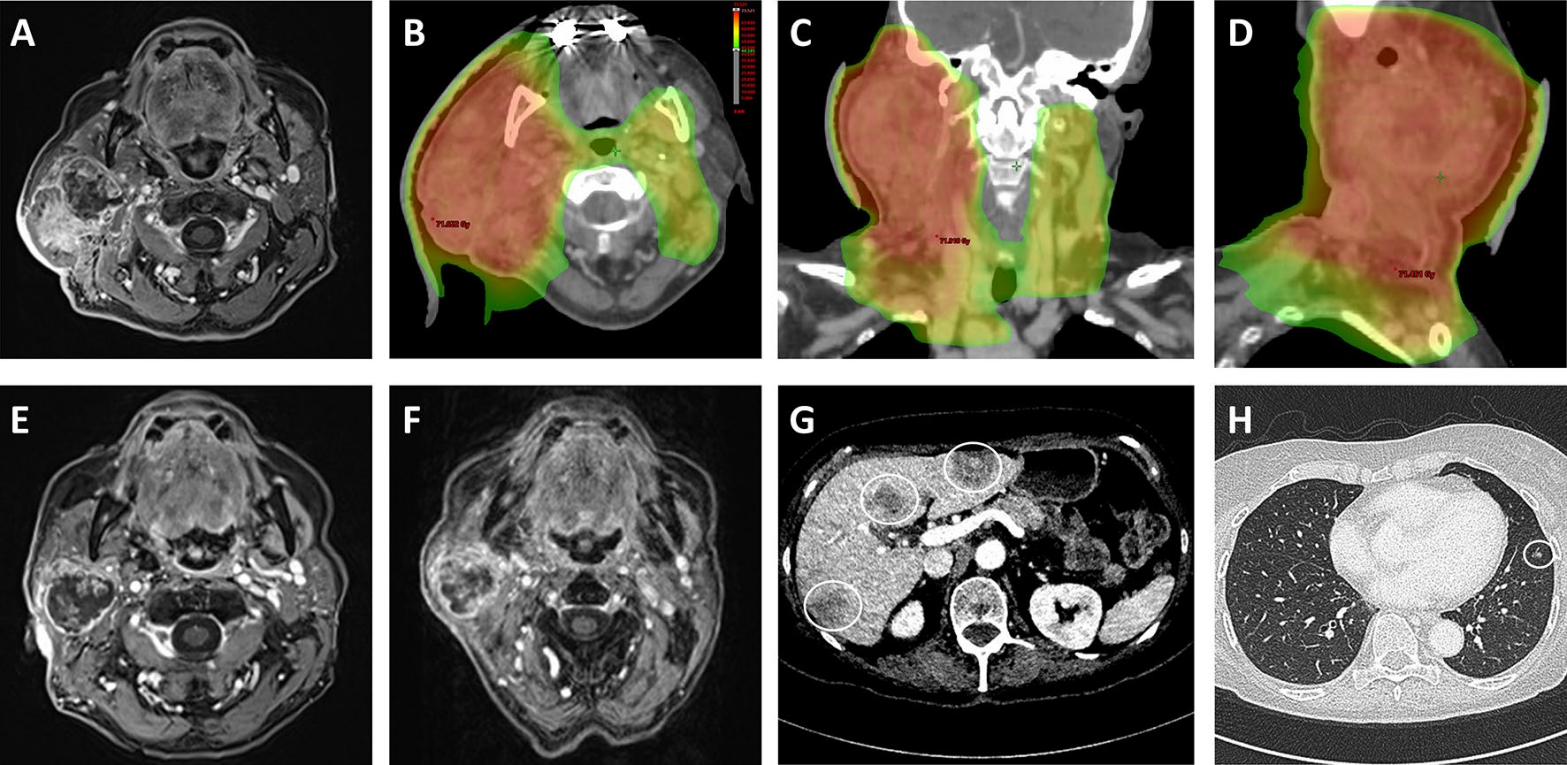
Definitive chemoradiotherapy for an undifferentiated (G4) parotid gland carcinoma in a 76-year-old patient. The locally advanced parotid gland carcinoma (cT4a cN2b cM0) was treated with intensity-modulated radiotherapy in a simultaneous-integrated boost-concept (SIB) between December 2019 and January 2020. While the high-risk PTV received 69.3 Gy delivered in 33 fractions, the medium-risk PTV and low-risk PTV were treated with 62.7 Gy and 56.1 Gy, respectively. a Pre-therapeutic contrast-enhanced T1-weighted MRI image in October 2019 demonstrating a right-sided parotid gland carcinoma infiltrating the cutis and the sternocleidomastoid muscle with ipsilateral lymph node metastases in level II, III, IV and V. b–d Radiotherapy treatment plan showing the dose distribution in an axial (b), coronary (c) and sagittal (d) view. e, f Follow-up images in March 2020 (e) and June 2020 (f) showing regression of the primary tumor and lymph node metastases with no signs for local or locoregional recurrence. g, h However, multiple hepatic hypodensities (g) and pulmonary noduli (h) suspicious for distant progression occurred in June 2020
